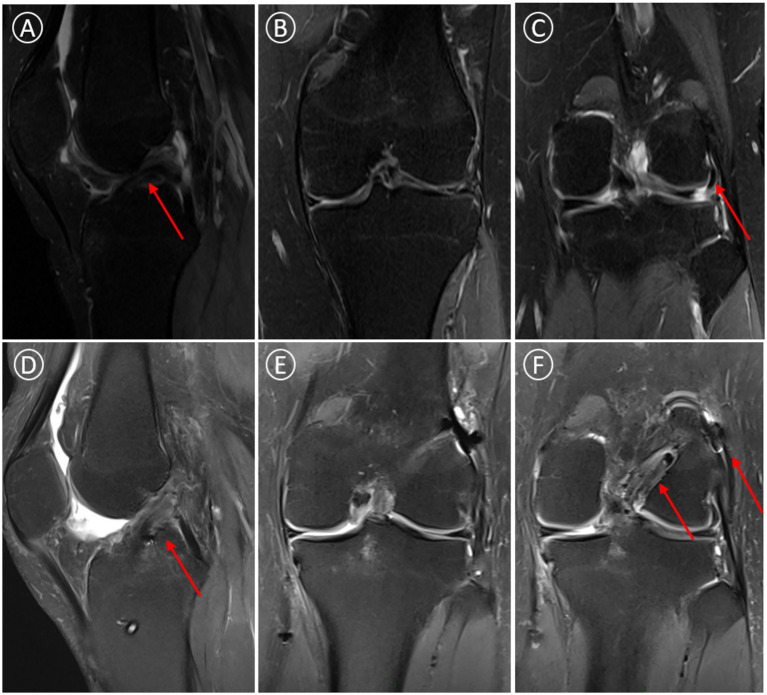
(A–C) Images show preoperative magnetic resonance images, (D–F) images show postoperative magnetic resonance images of an 18-year-old girl captured at 12 months.
